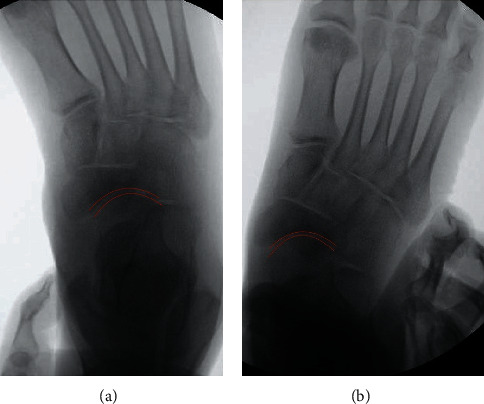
(a) 12-week follow-up with adduction stress of the forefoot. Intraoperative images of the foot showing normal navicular and talonavicular joint radiography and stability with real-time stress radiographs after complete healing and hardware removal; red lines demonstrate the joint surface of the talus and navicular at the talonavicular joint. (b) 12-week follow-up without adduction stress of the forefoot. Intraoperative images of the foot showing normal navicular and talonavicular joint radiography and stability with real-time stress radiographs after complete healing and hardware removal; red lines demonstrate the joint surface of the talus and navicular at the talonavicular joint.
